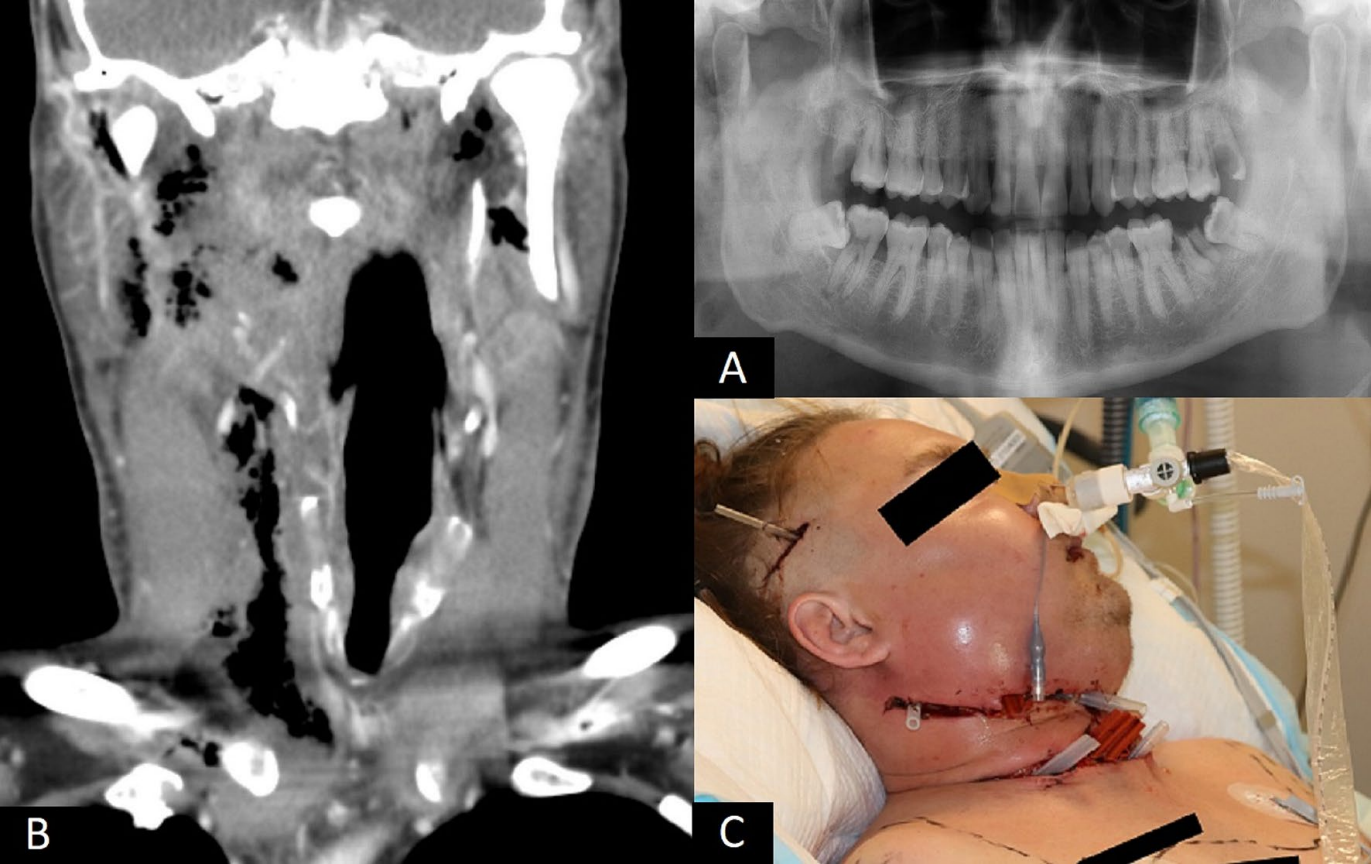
A 40-year-old male smoker without underlying diseases had had toothache in the right lower jaw for 3 days before onset of significant swelling and fever (39.2 °C). At hospital admission, C-reactive protein level was 253 mg/L and white blood cell count was 18.2 10^9^/L. The patient underwent three wide surgical interventions to subside the deep oral, facial, and neck infection. Bacterial culture from the pus sample showed *Streptococcus anginosus* and mixed anaerobic flora. Blood culture was also positive (anaerobic Gram-positive coccus and anaerobic Gram-negative rod). A. Dental panoramic tomography radiograph showed the right mandibular third molar with periapical lesion as the main infection focus. B. Computer tomography imaging at hospital admission demonstrated bilateral gas formation in neck tissues from the level of skull base to the upper mediastinal space at the right side. The airway was also deviated. C. Extensive neck approaches were required, including temporal and upper mediastinal drainages. Swelling and tightness in buccal and periorbital areas remained significant after the third surgery. The overall length of hospital stay was 32 days. The patient eventually recovered well from the infection. A written consent was obtained from the patient for use of the image
